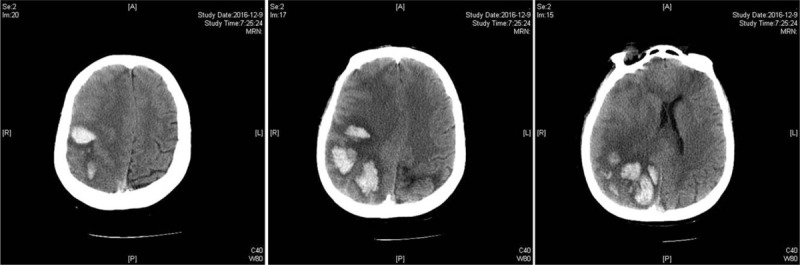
On the fourth day of hospitalization, CT reexamination demonstrated multiple patchy high-density shadows in the right occipital and parietal lobes. CT = computed tomography.
